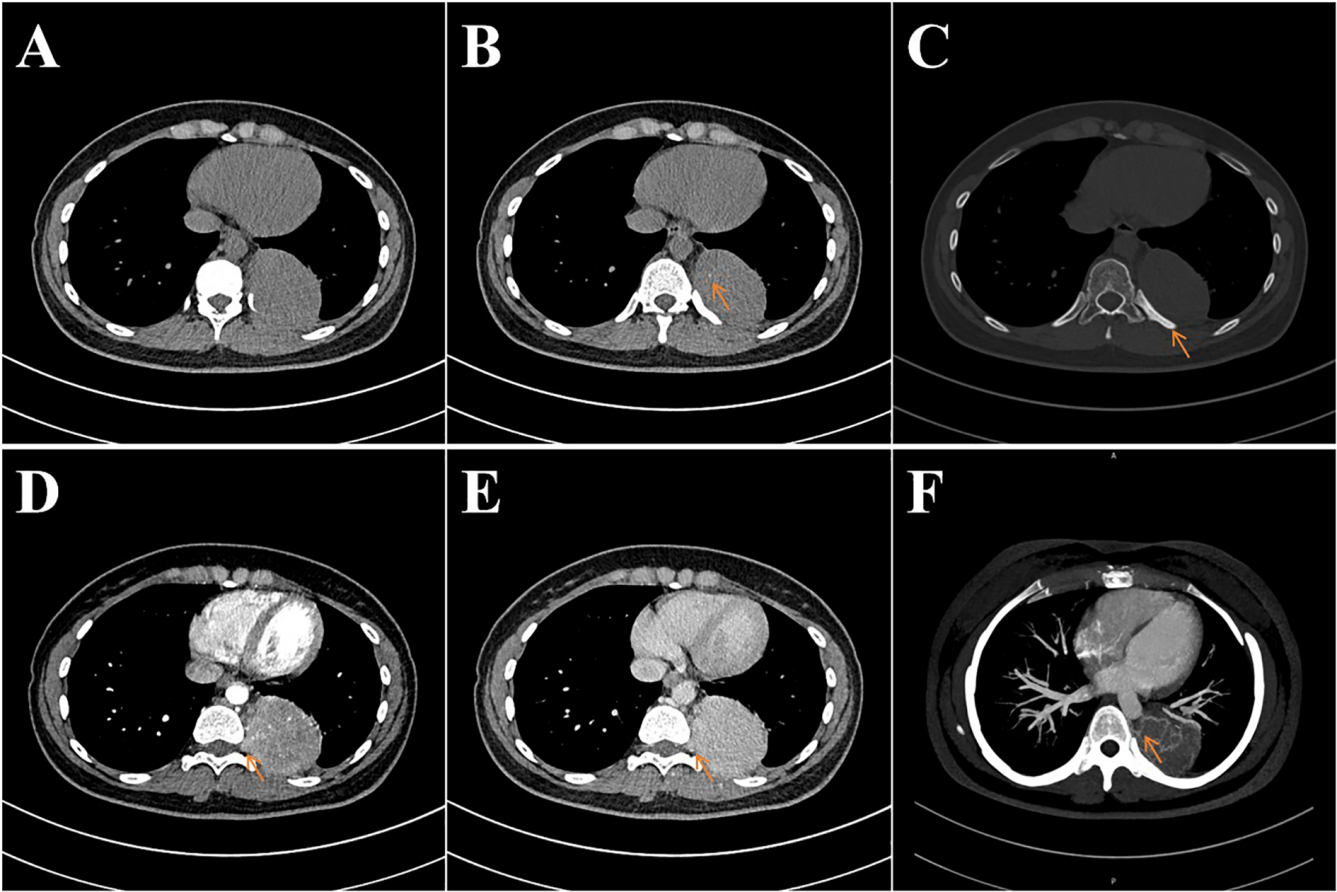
The patient’s plain and contrast-enhanced CT images. (A) shows a well-defined soft tissue density mass at the left posterior mediastinal border. (B) demonstrates fine punctate calcifications within the lesion. (C) reveals increased bone density in the adjacent ribs. (D, F) indicate involvement of the left T9 intervertebral foramen region, with persistent marked enhancement after contrast administration. (F) demonstrates thickened and tortuous feeding arteries (originating from the thoracic aorta) both peritumorally and intratumorally.
